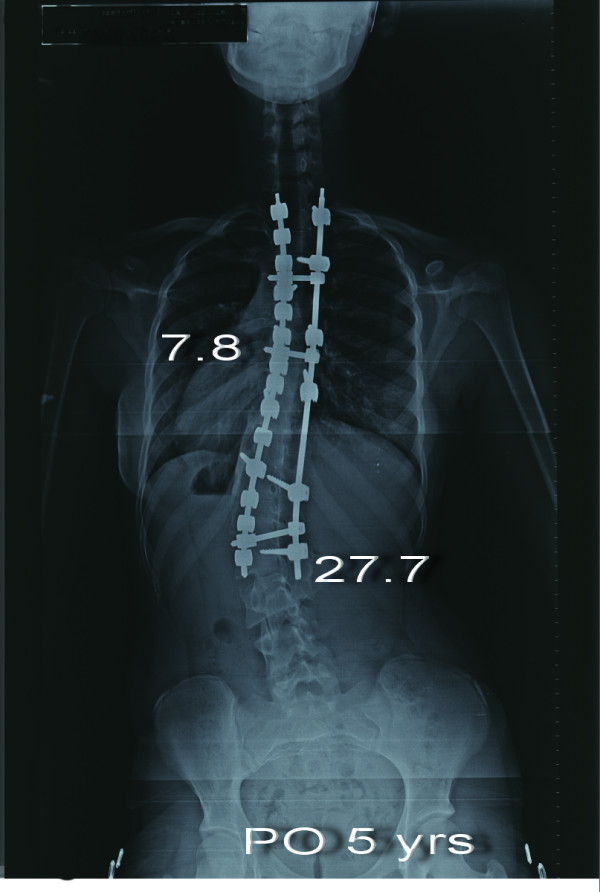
Anteroposterior radiograph of the patient taken 5 years after surgery. The thoracic curve was 7.8° and the lumbar curve was 27.7°. Increase of vertebral tilt in distal segments is notable.
